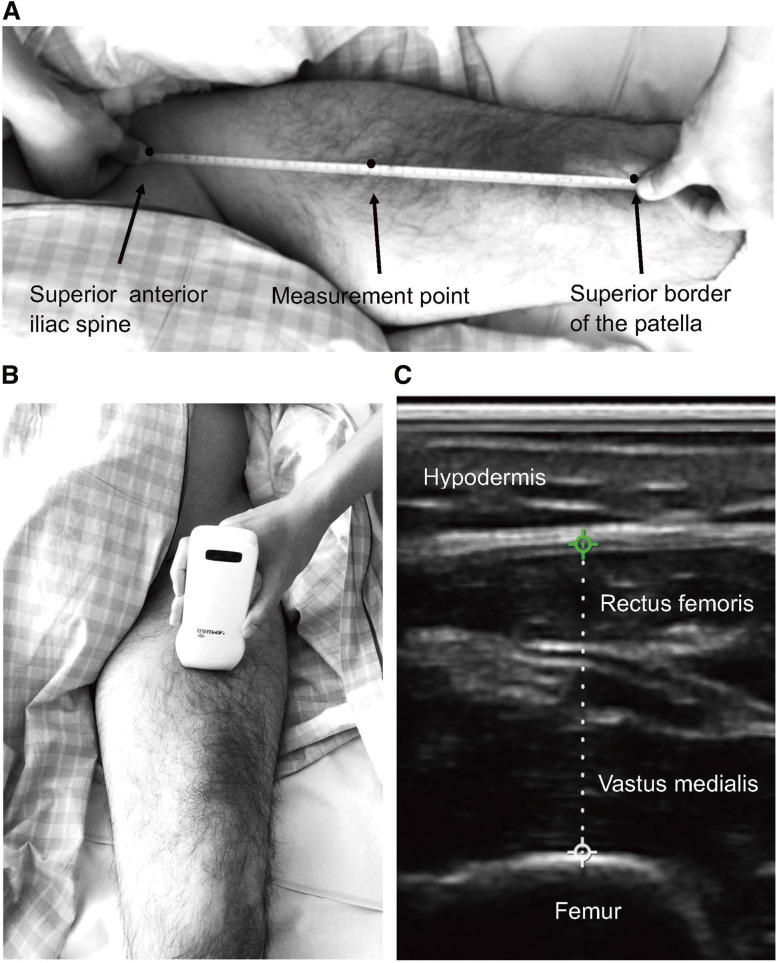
Ultrasound image of representative quadriceps muscle thickness with probe placement and measurement method (A) The measurement site. (B) The location of the probe. (C) A digital tape measure built into the US device was used to measure the thickness of the rectus femoris and vastus medialis muscles located directly above the superior border of the femur. The dotted line indicated by the digital tape measure shows the combined muscle thickness of the rectus femoris and vastus medialis muscles
